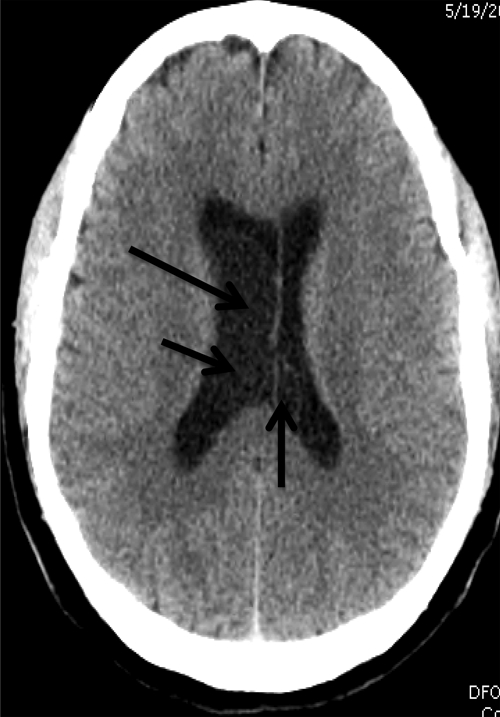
Computed tomography of the head of the patient without contrast showing two right intraventricular and one left intraventricular cystic lesions.
